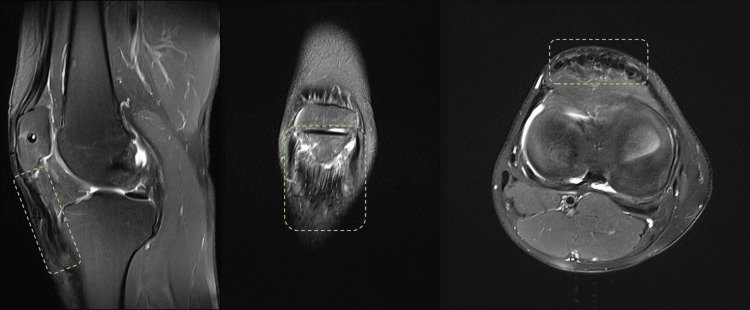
One-year follow-up MRI (green dashed line indicating full integration of the reconstruction)
